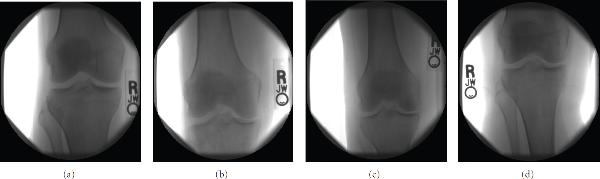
X-ray images of four different KL grades: (a) 0 (normal), (b) 1 (doubtful), (c) 2 (mild), (d) 3 (moderate).
